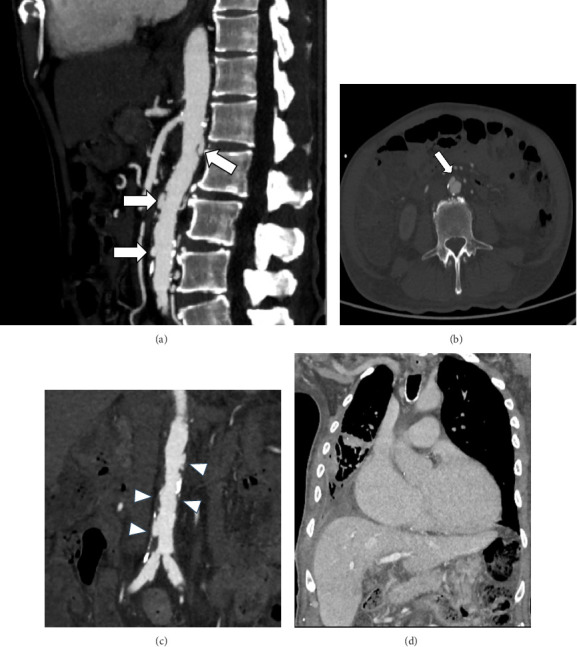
(a) CTA abdominal aorta in sagittal view shows multiple small aneurysms (white arrows). (b) CTA abdominal aorta in axial view shows multiple small aneurysms (white arrows). (c) CTA abdominal aorta in coronal view demonstrates features of aortitis involving the abdominal aorta and bilateral common iliac arteries (white arrows). (d) CECT thorax in coronal view shows cardiomegaly with consolidation of the right lower lobe suggestive of pneumonia.
